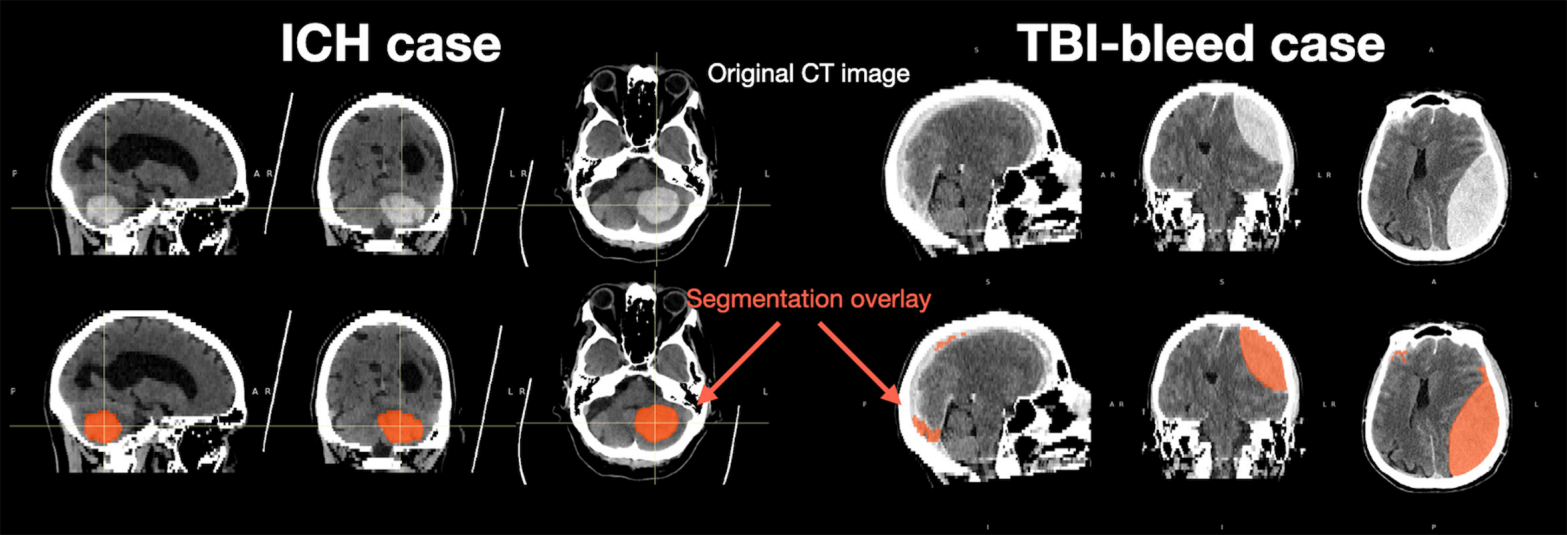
Representative data from ICH (left) and TBI-bleed (right) cases. The original CT images are shown in the top row and the bottom row shows the Viola-AI-based segmentation in red.
